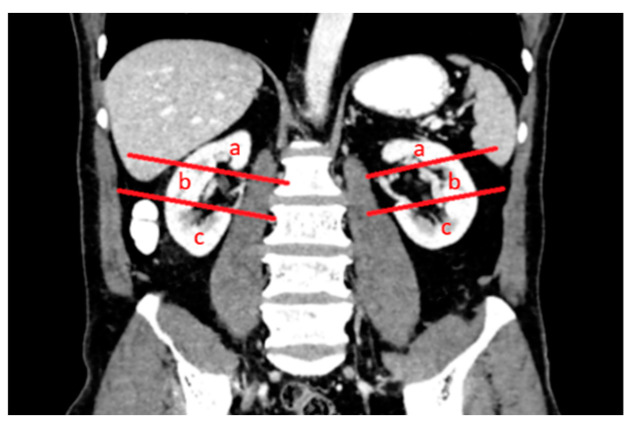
Polar lines (red lines) parallel to the hilar vascular structures of both kidneys were identified in the coronal venous-phase CT image. The upper, middle, and lower pole locations of the lesions were determined according to the upper and lower polar lines and the location of the lesions was according to the L component of the R.E.N.A.L. nephrometry scoring: (a) upper pole, (b) middle pole and (c) lower pole.
